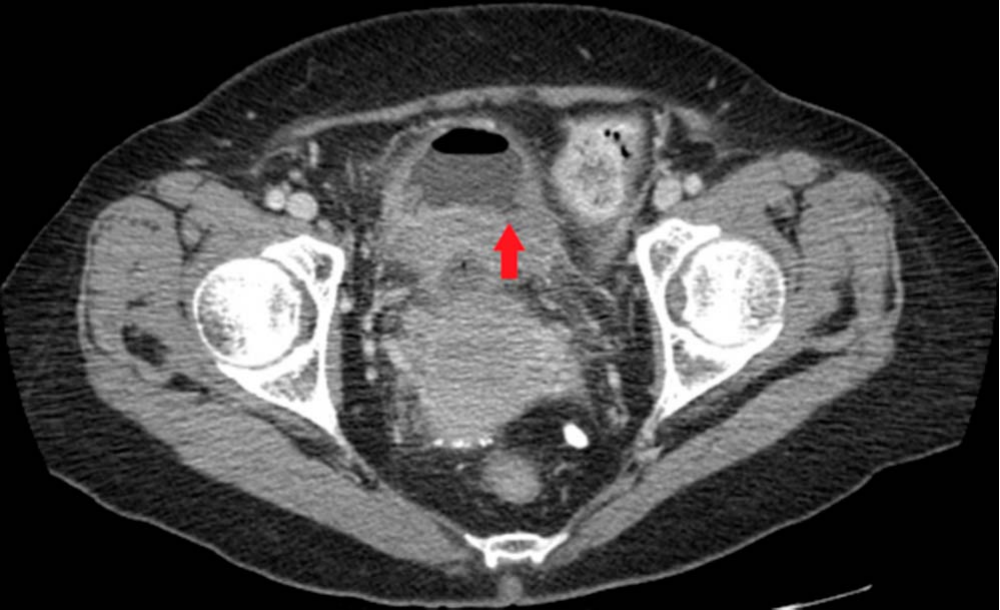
Computed tomography showing bladder wall thickening (red arrow).
